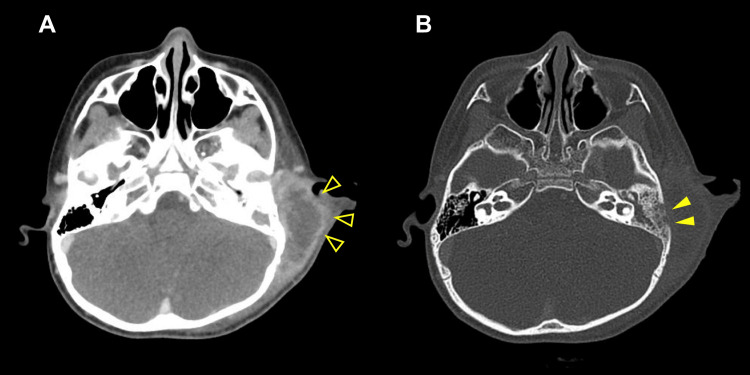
Contrast-enhanced CT In the left auricular bone protrusion, (A) loss of air in the auricular cavity, a low-density area suggesting abscess formation, and (B) bone dissolution were observed. CT: computed tomography
